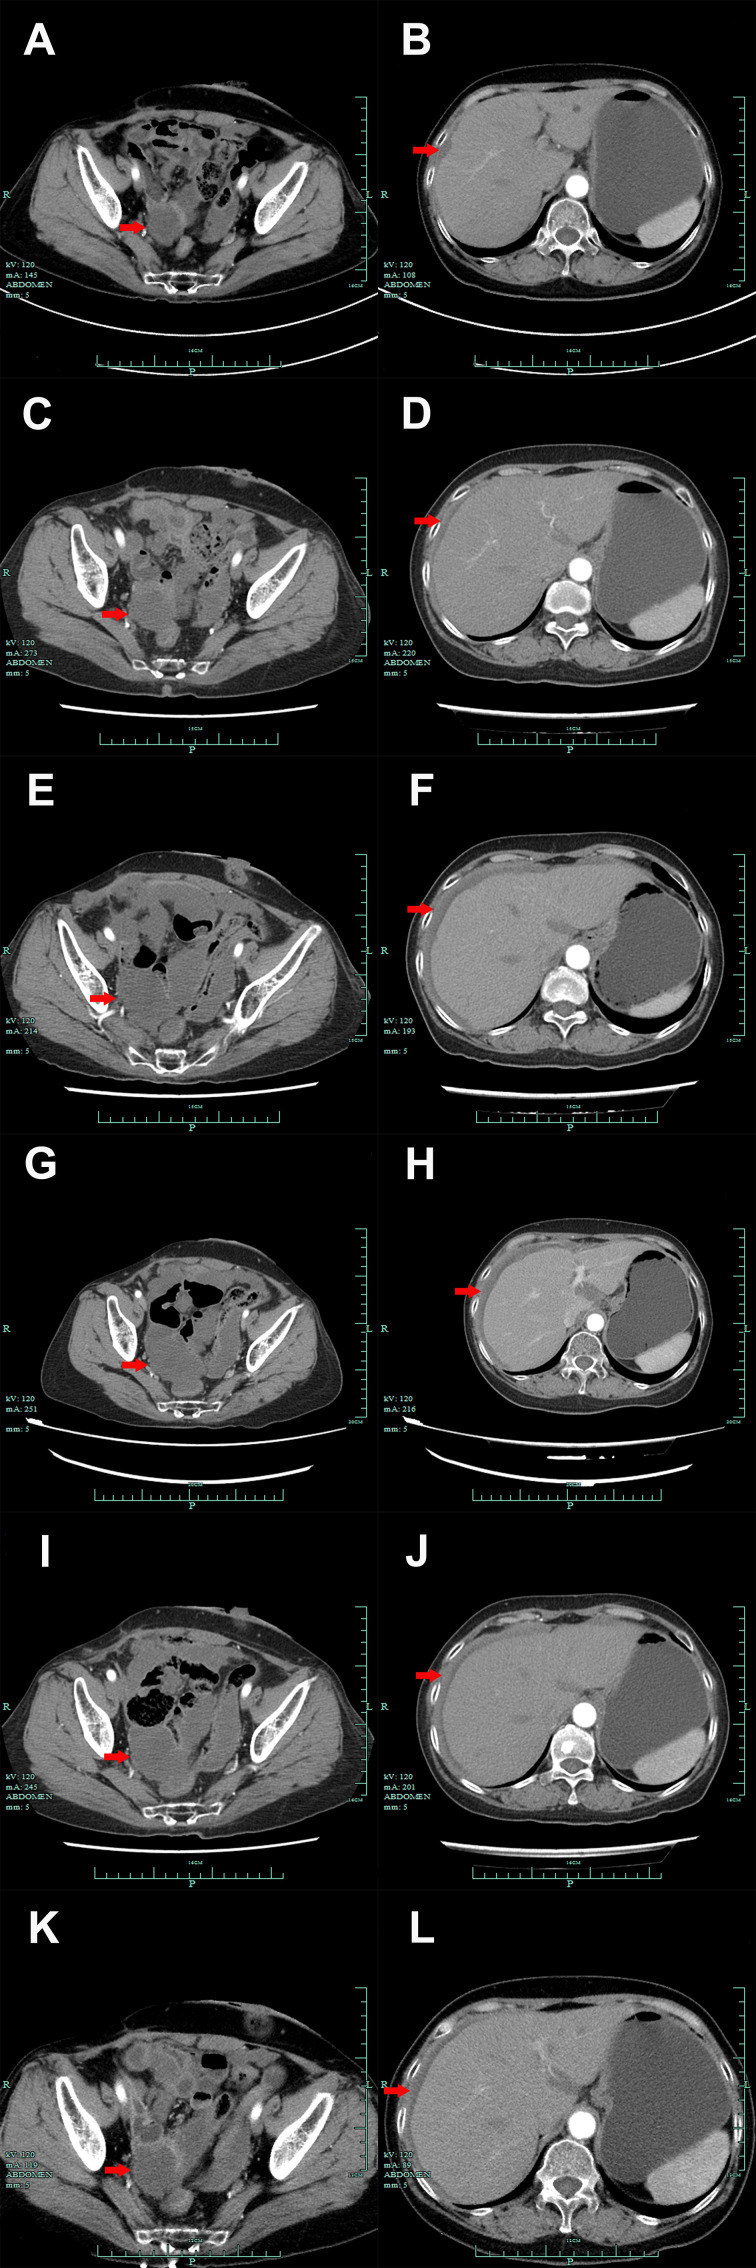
Abdomen CT scan presentations suggested the emerging occupation behind the uterus (A, C, E, G, I, K, red arrow) and thickened peritoneum (B, D, F, H, J, L, red arrow) from October 2020 to March 2022. (A, B). October 2020. (C, D). December 2020. (E, F). January 2021. (G, H). May 2021. (I, J). September 2021. (K, L). March 2022.
